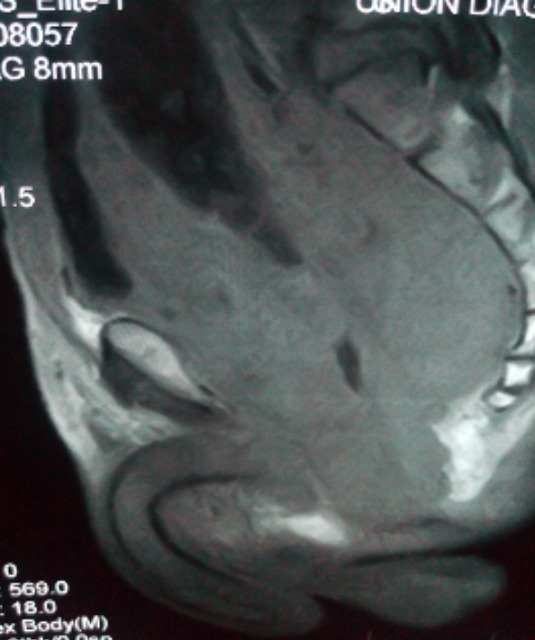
Lateral pelvic Xray showing large bowel obstruction from PCa
